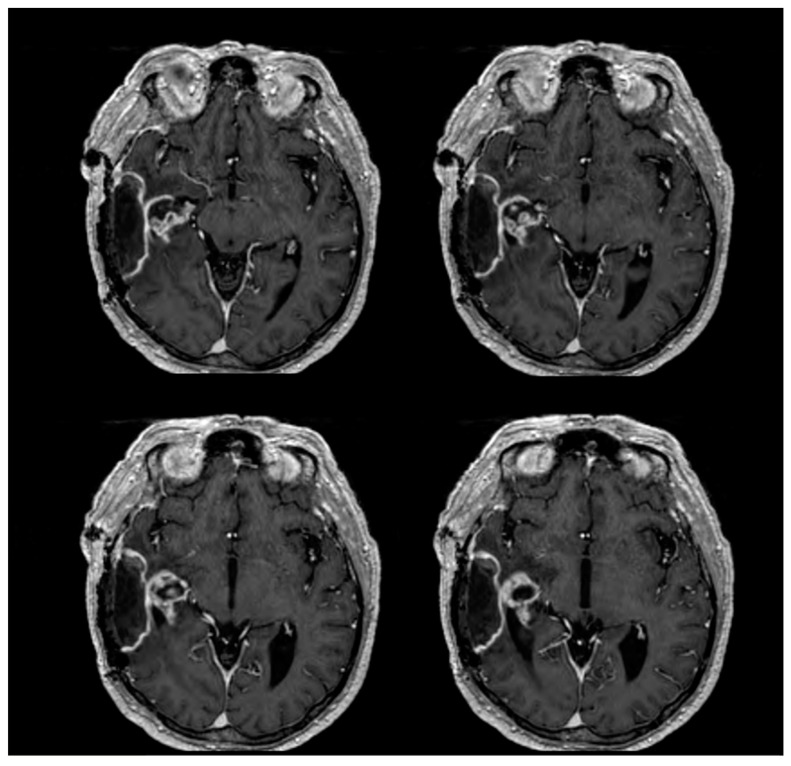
Postoperative T1-weighted brain MRI axial sequences with gadolinium demonstrate the complete removal of the larger lesion and the stability of the smaller temporo-mesial right lesion.
